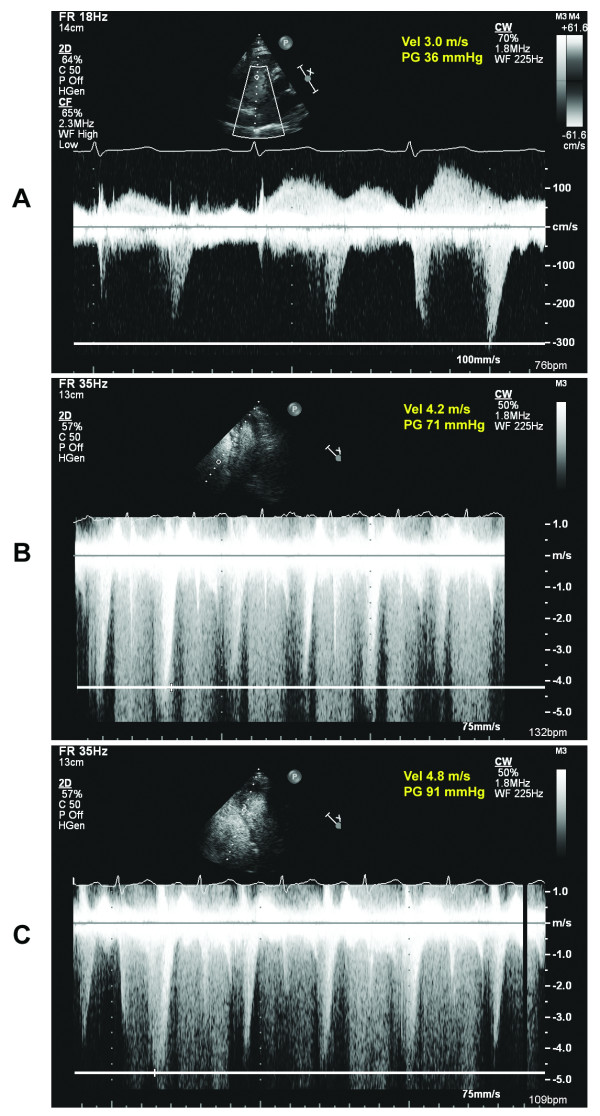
Continuous wave Doppler across tricuspid valve at rest (A), stress (B) and recovery (C). The estimated PASP (ΔP = 4V^2^+RAP, where V is the tricuspid regurgitation velocity and RAP is the estimated right atrial pressure of 10 mmHg) is 46 mmHg at rest, 81 mm Hg during stress and 101 mm Hg immediately post stress.
